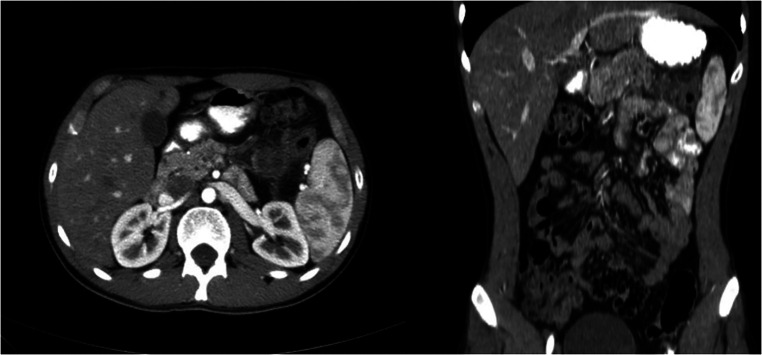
CT scan (axial and coronal) cuts showing lobulated hypo-dense lesion in the pancreatic tail, measuring 3 cm × 2.5 cm with minimal rim enhancement contains at least three cysts, the largest measuring 1.5 cm with stranding of the adjacent mesenteric fat.
